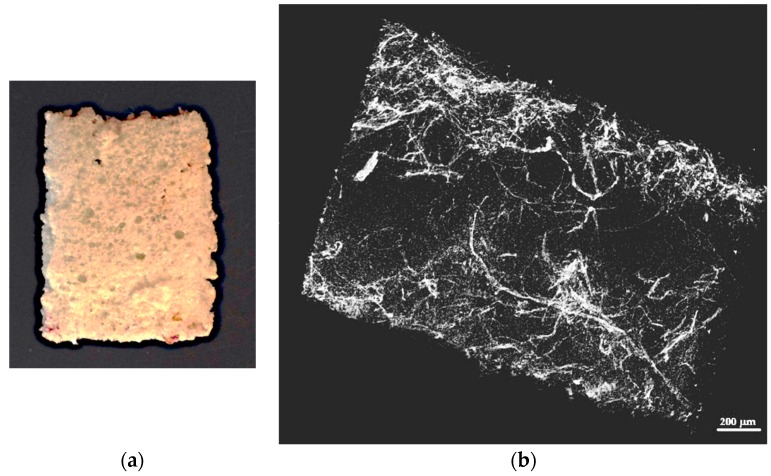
(a) Optical; and (b) X-ray tomography image of the polyurethane foam with 9% weight fraction of MDF fibers.
